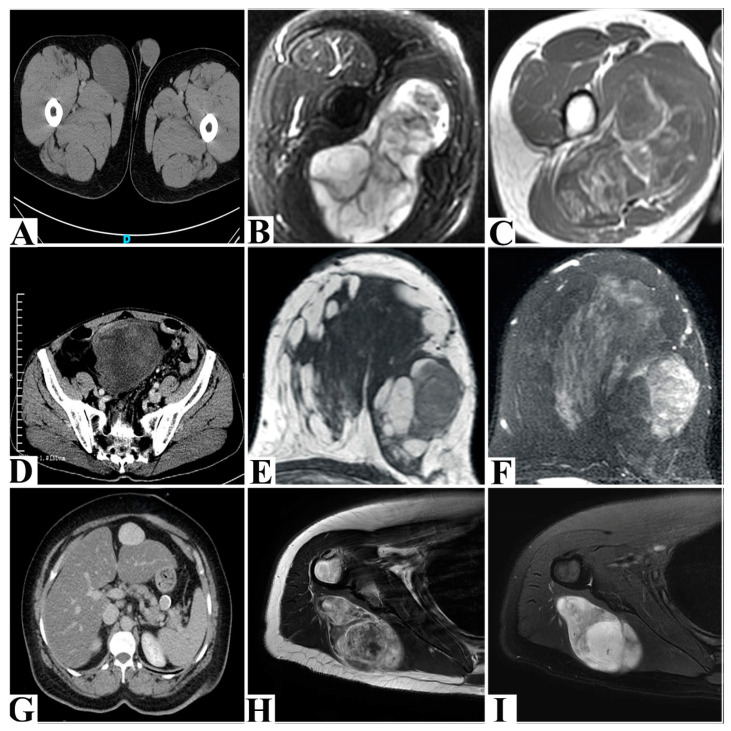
(A) Computed tomography shows a myxoid liposarcoma in the medial part of the subcutaneous soft tissues of the right thigh, measuring 7.3 × 5.6 cm. Adapted from Correa, N. et al. (2026) [33], licensed under CC BY 4.0. (B) Myxoid liposarcoma in axial T1 sequence reveals hypointensity of the lesion. Adapted from Natella, R. et al. (2023) [22] and licensed under CC BY 4.0. (C) Myxoid liposarcoma in axial T2 sequence, with fat suppression, shows hyperintensity of the lesion. Adapted from Natella, R. et al. (2023) [22] and licensed under CC BY 4.0. (D) Contrast-enhanced computed tomography of a pleomorphic liposarcoma located in the lower abdomen with a heterogeneous appearance and clear boundaries. Adapted from Wang, L. et al. (2018) [37] and licensed under CC BY 4.0. (E) Pleomorphic liposarcoma in axial T1-weighted image reveals heterogeneous areas and areas of hyposignals. Adapted from Jo, S.J. et al. (2020) [40] and licensed under CC BY 4.0. (F) Pleomorphic liposarcoma in axial T2-weighted image shows heterogeneous areas and areas of hypersignal. Adapted from Jo, S.J. et al. (2020) [40] and licensed under CC BY 4.0. (G) Contrast-enhanced computed tomography revealed a myxoid pleomorphic liposarcoma as a well-defined mass with progressive contrast, located anterior to the left hepatic lobe. Adapted from AlObaid, B. et al. (2022) [44] and licensed under CC BY 4.0. (H) Myxoid pleomorphic liposarcoma examined by T1-weighted magnetic resonance imaging showing a heterogeneous soft tissue mass with a myxoid component in the teres minor muscle. Adapted from Choi, J.H. et al. [49] and licensed under CC BY 4.0. (I) Myxoid pleomorphic liposarcoma was examined by T2-weighted fat-suppressed magnetic resonance imaging, showing a heterogeneous soft tissue mass with a myxoid component in the teres minor muscle. Adapted from Choi, J.H. et al. [49] and licensed under CC BY 4.0.
